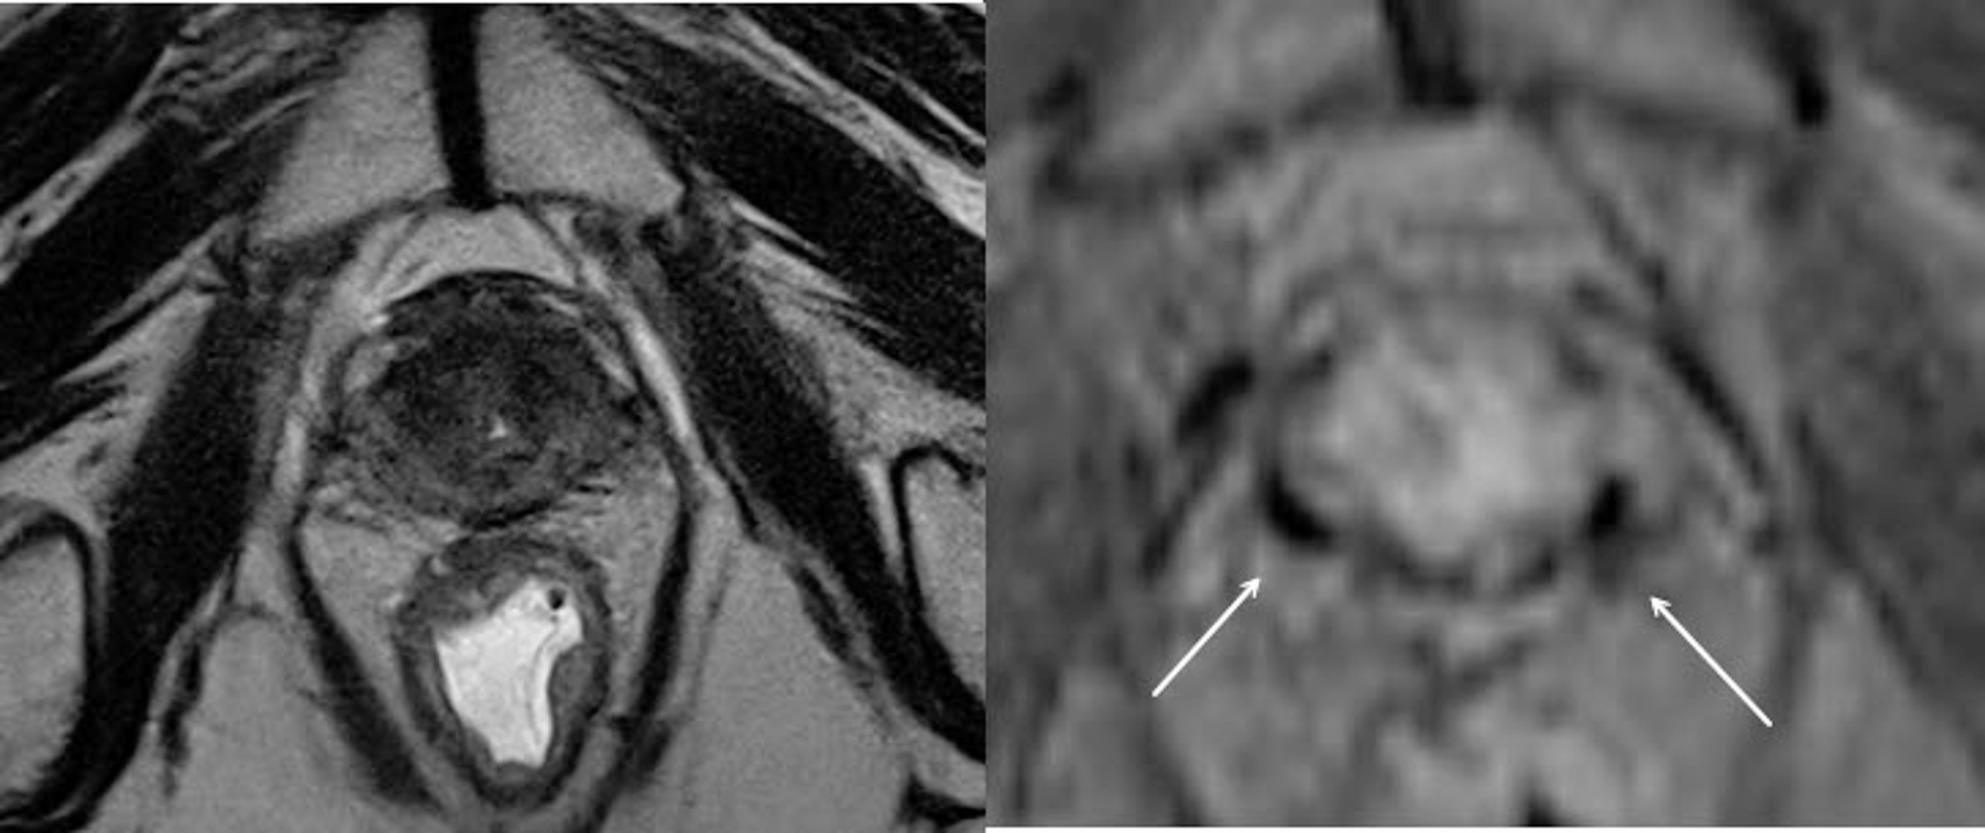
Prostate MRI before biopsy. Left: T2-weighted sequence; right: T1-weighted sequence. The two white arrows indicate the two hypointense gold markers
